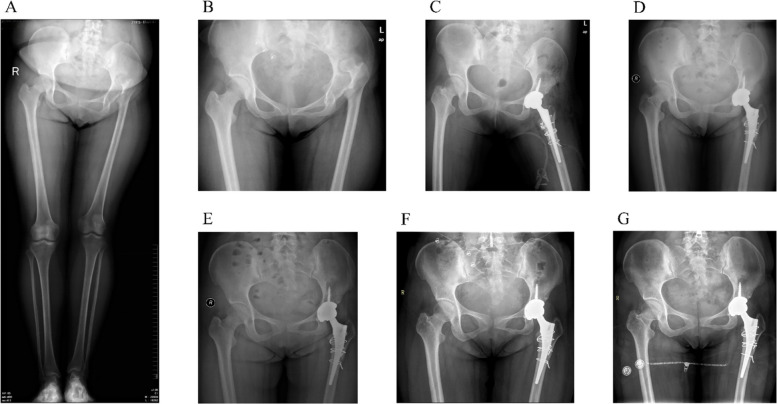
Serial radiographs of a 28-year-old woman with left-sided Hartofilakidis type C developmental dysplasia of the hip before and after total hip arthroplasty. A Preoperative full-length standing radiograph showing left high hip dislocation with a leg-length discrepancy of 3.2 cm. B Preoperative anteroposterior pelvic radiograph demonstrating the dysplastic acetabulum and proximally migrated femoral head. C Anteroposterior pelvic radiograph on postoperative day 1 showing the acetabular component placed at the anatomical hip centre. D Anteroposterior pelvic radiograph at 3 months postoperatively showing progressive consolidation at the femoral osteotomy site. E Anteroposterior pelvic radiograph at 6 months postoperatively showing complete osteotomy union (acetabular inclination 41.2°, acetabular anteversion 19°, femoral offset 3.21 cm, residual leg-length discrepancy 0.44 cm). F and G Anteroposterior pelvic radiographs at 12 and 24 months postoperatively, respectively, demonstrating stable implant fixation without evidence of stem subsidence, component loosening, or periprosthetic osteolysis
